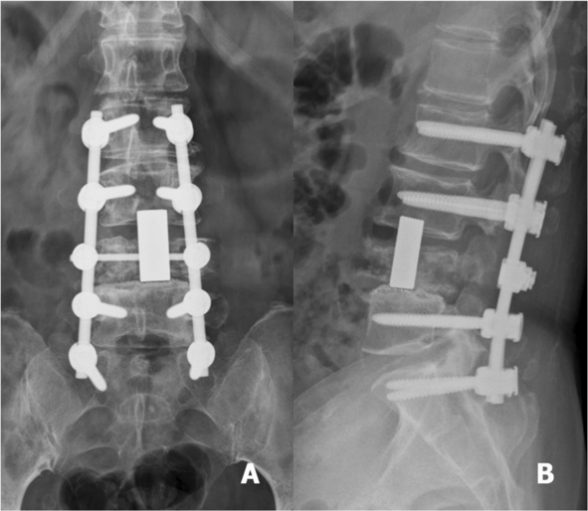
Post-operative anteroposterior (a) and lateral (b) radiographs revealed that the vertebral body and tumour were removed. Good correction of spinal alignment was achieved by cervical TM mesh cage implantation and posterior pedicle screws fixation
